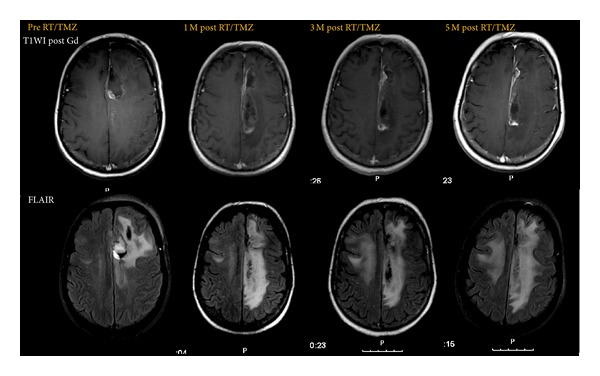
T1-contrast-enhanced magnetic resonance imaging (MRI) documenting pseudoprogression according to Macdonald criteria showing a significant increase in cingulate gyrus enhancing lesion at 1 month after RT/TMZ with subsequent stabilization (stable corticosteroid dose and functional status). FLAIR sequence shows increased signal extending to contralateral hemisphere anticipating true progressive disease.
